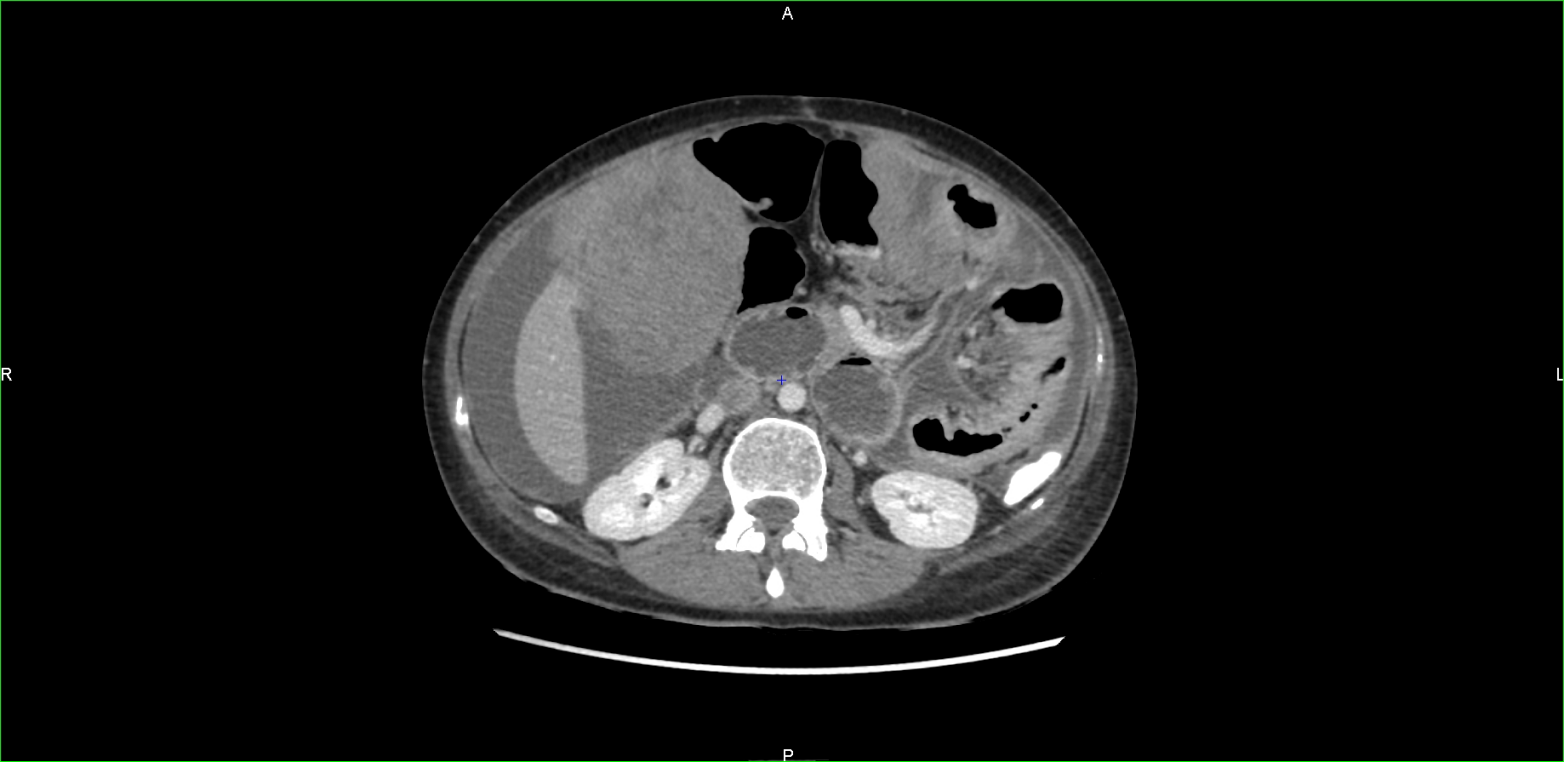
CT scan showing early and massive relapse.
